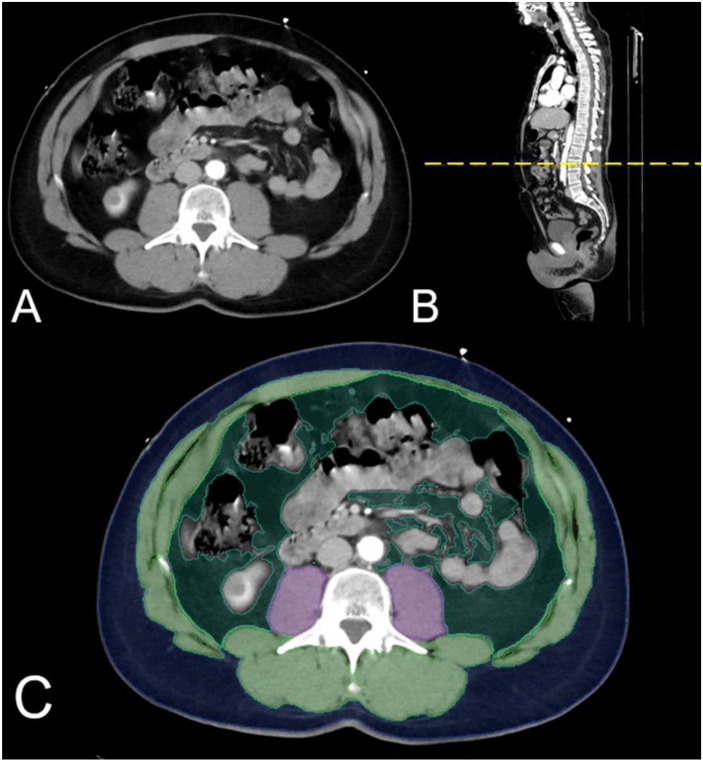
AI based CT Image Segmentation. (A,B): Example Original axial & sagittal CT images at the level of the third lumbar vertebra. (C): CT image with automated tissue segmentation.
